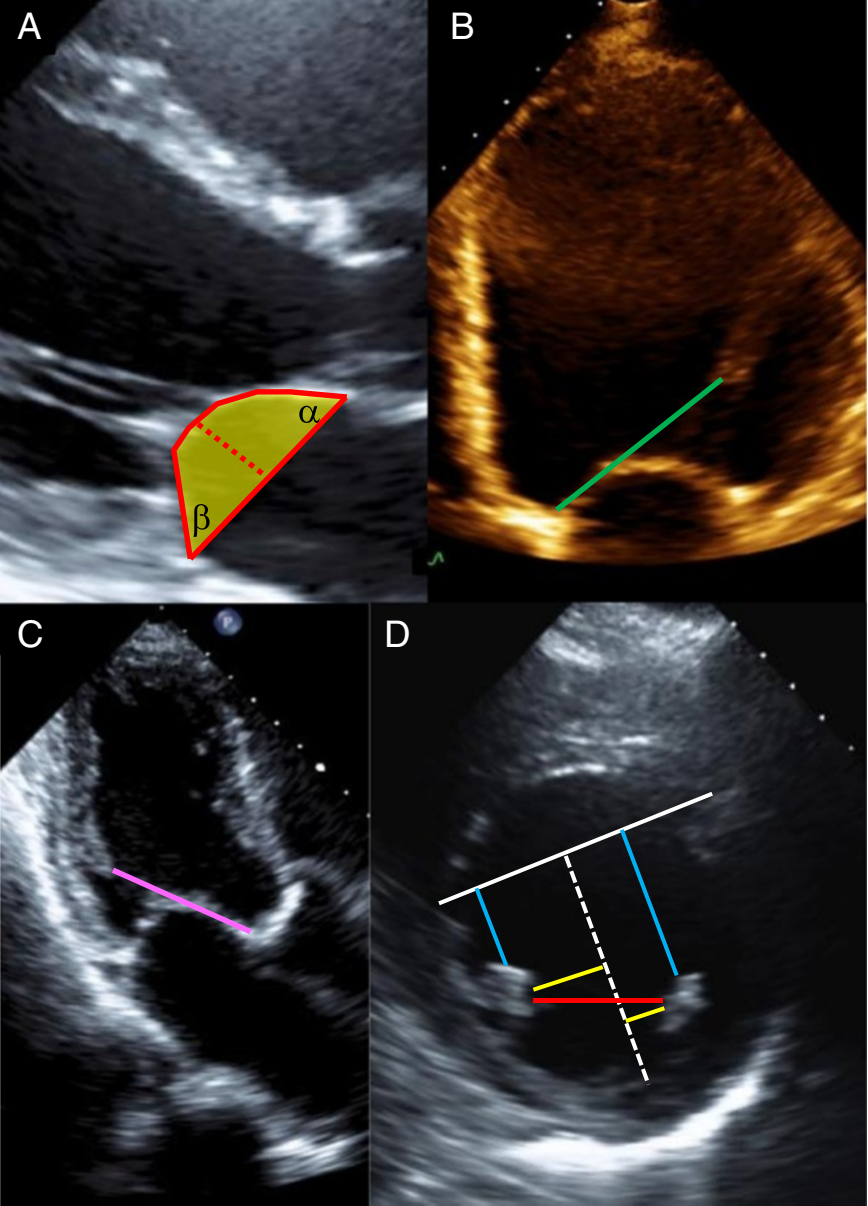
Representative measurements of tenting height, tenting angles, and tenting area. Panel A shows the measurement of tenting height, tenting area, and tenting angles in a mid-systolic parasternal long axis TTE image. The mitral annulus line is drawn and the distance from the annulus line to the coaptation point (red hatched line) represents tenting height. The posterior and anterior leaflets’ silhouettes are traced from the annular line to the coaptation zone to delineate an area (yellow shading) that represents the tenting area. Tethering angles are measured as the angle that the mitral leaflets create with the mitral annulus line. Panel B shows measurement of apical displacement the anterolateral PM in the apical four chamber view (green line), and Panel C shows measurement of apical displacement the posteromedial PM in the apical three chamber view (pink line). Panel D shows a representative parasternal short-axis mid-ventricular level view in early systole with PM bodies in cross-section. A reference grid of a mid-septal perpendicular chord (white line) spanning the septal insertions of right ventricle myocardium and another line orthogonal to and originating from the center of the first line (hatched white line) was created. The mid-septal perpendicular chord allows posterior displacements of both PMs to be measured (blue lines), as well as to measure lateral displacements of both PMs (yellow lines). Intra-PM distance is shown by the red line. These distances are all elevated in CIMR compared to normal controls. Though there is significant overlap in the distances among symmetric and asymmetric CIMR phenotypes, relative displacements of the posteromedial PM are increased in asymmetric CIMR as compared to the anterolateral PM (see Table 5).
